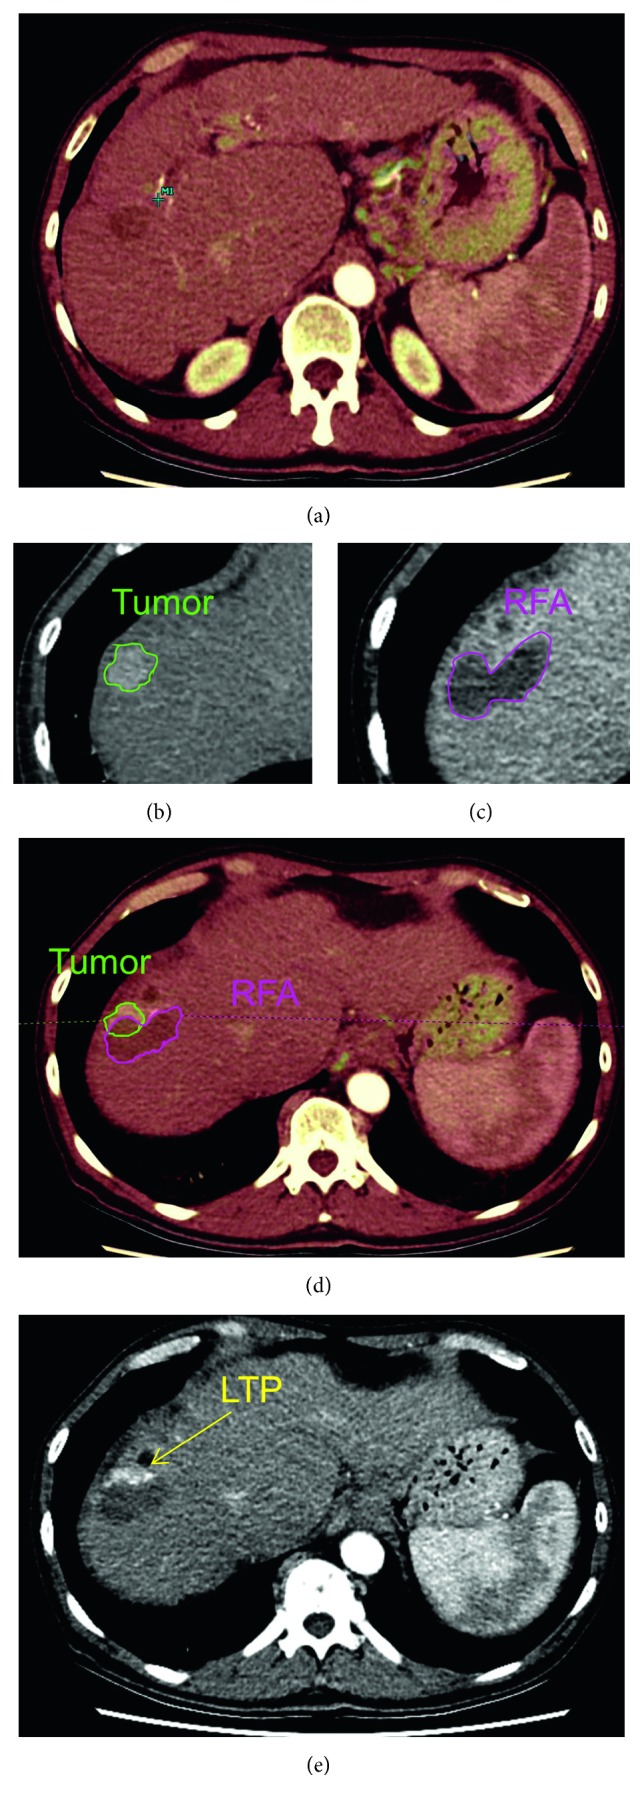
Image analysis protocol. (a) Registration (overlay) of preinterventional and postinterventional CT scans. (b) Semiautomatic delineation of tumor volume. (c) Semiautomatic delineation of RFA volume. (d) Image fusion plane: margin analysis by overlaying pre- and postinterventional imaging. (e) Follow-up scan with local tumor progression.
